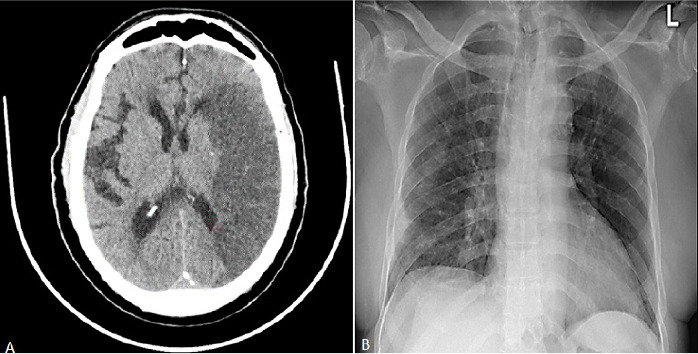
(A) non-contrast CT Head demonstrates left middle cerebral artery territory infarct with pressure effect effacing cortical sulci and ipsilateral lateral ventricle anterior horn; (B) AP chest X-ray revealed features of right lung pneumonia seen as patchy peripheral faint ground glass shadowing in the right lower zone
